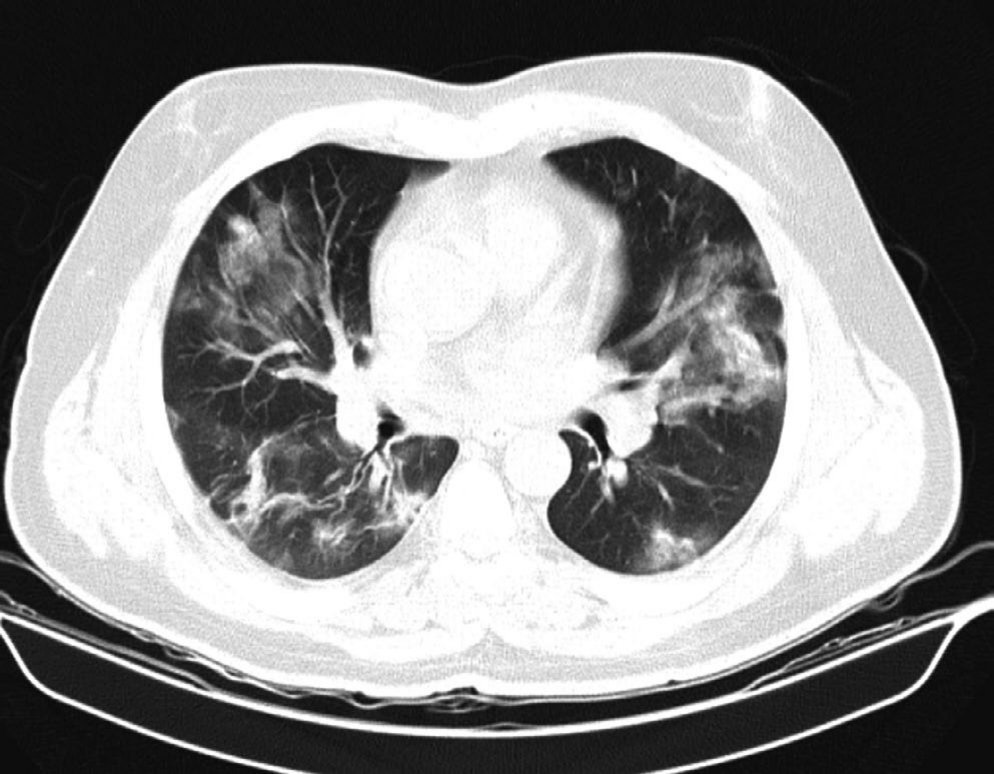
Chest CT scan revealed multifocal peribronchial ground‐glass opacities and consolidation at first admission
